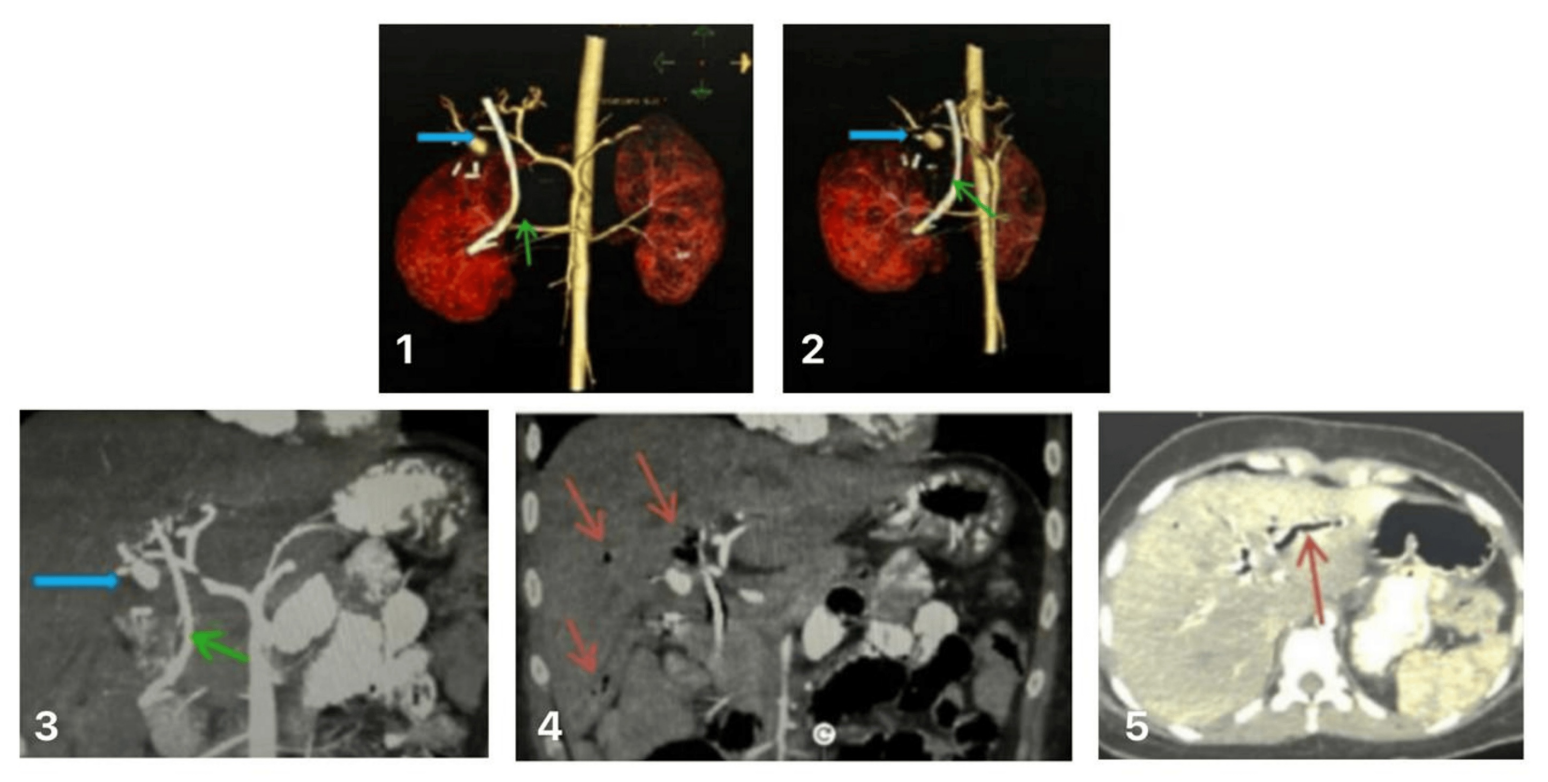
Computed tomography angiography (CTA) with 3D vascular reconstruction consistent with a pseudoaneurysm of the right hepatic artery branch Images 1-2: hepatic artery pseudoaneurysm (blue arrow); rounded, well-defined structure (11x14x15 mm) with a small filiform pedicle connected to the right hepatic artery, 20 mm from its origin Image 3: biliary stent (green arrow); proximal end in the common hepatic duct, distal end in the ampullary region of the common bile duct Images 4-5: biliary dilation and pneumobilia (red arrows) are likely due to recent instrumentation (endoscopic Retrograde cholangiopancreatography) and surgery (laparoscopic cholecystectomy)
